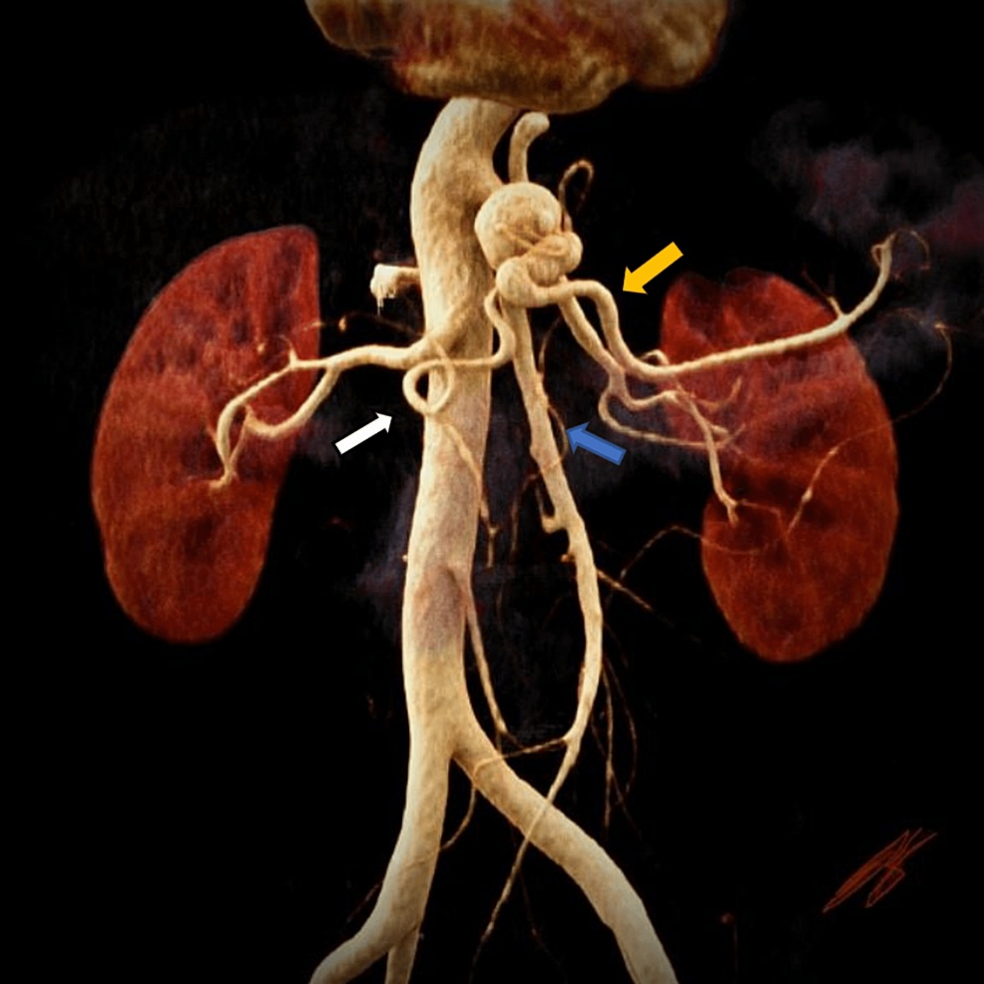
Pre-operative 3D reconstruction and cinematic rendering of the CTA with celiomesenteric trunk aneurysm denoting the splenic artery (orange arrow), hepatic artery (white arrow), and superior mesenteric artery (blue arrow) CTA: computed tomography angiography
